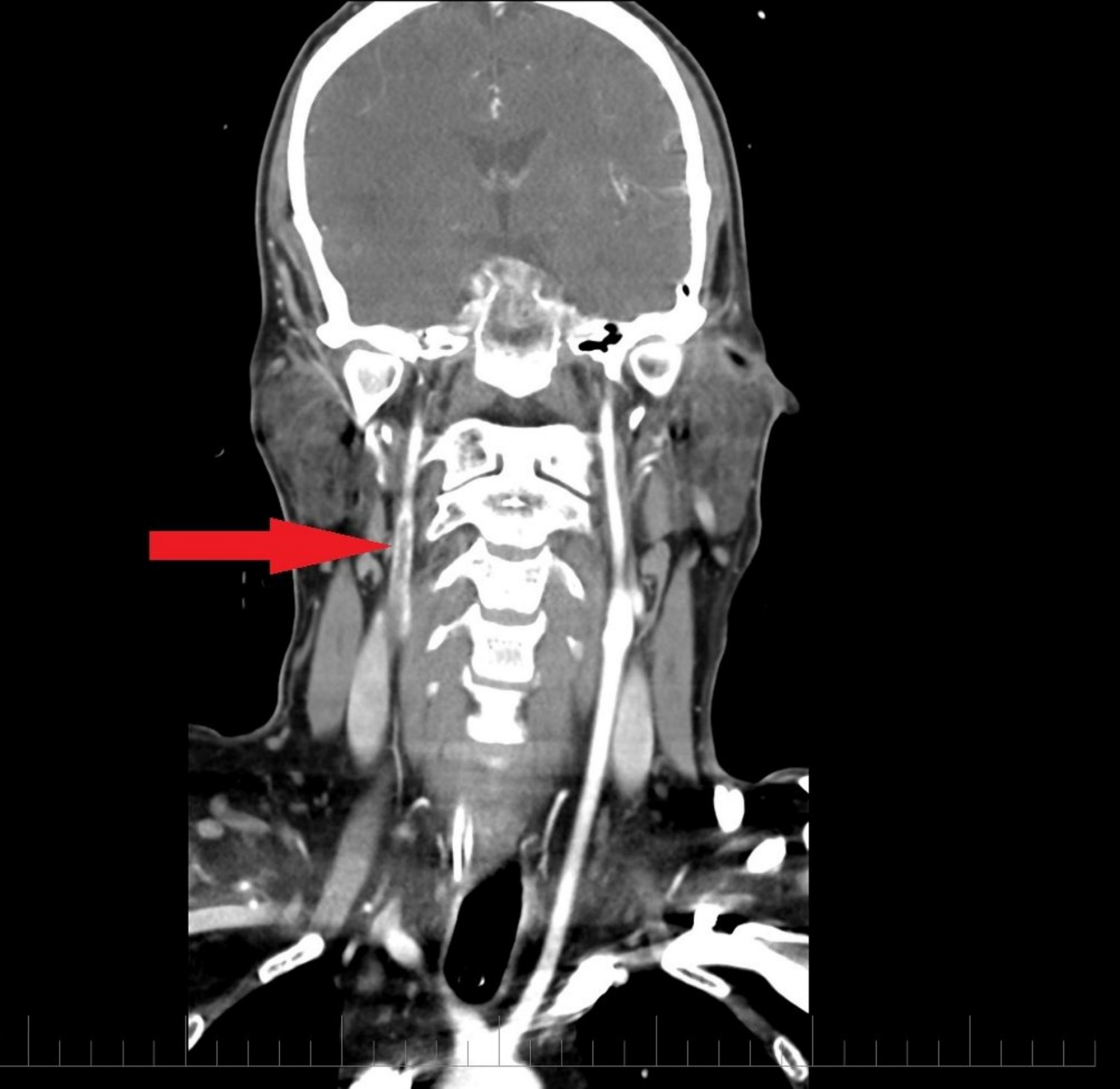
CT angiogram neck (longitudinal section) showing nonocclusive thrombus within proximal right internal carotid artery. CTA neck (longitudinal section) demonstrates a hypodense filling defect (red arrow) suggestive of nonocclusive thrombus in the proximal right internal caortid artery just distal to the bifurcation of the common carotid artery CTA: computed tomography angiogram
